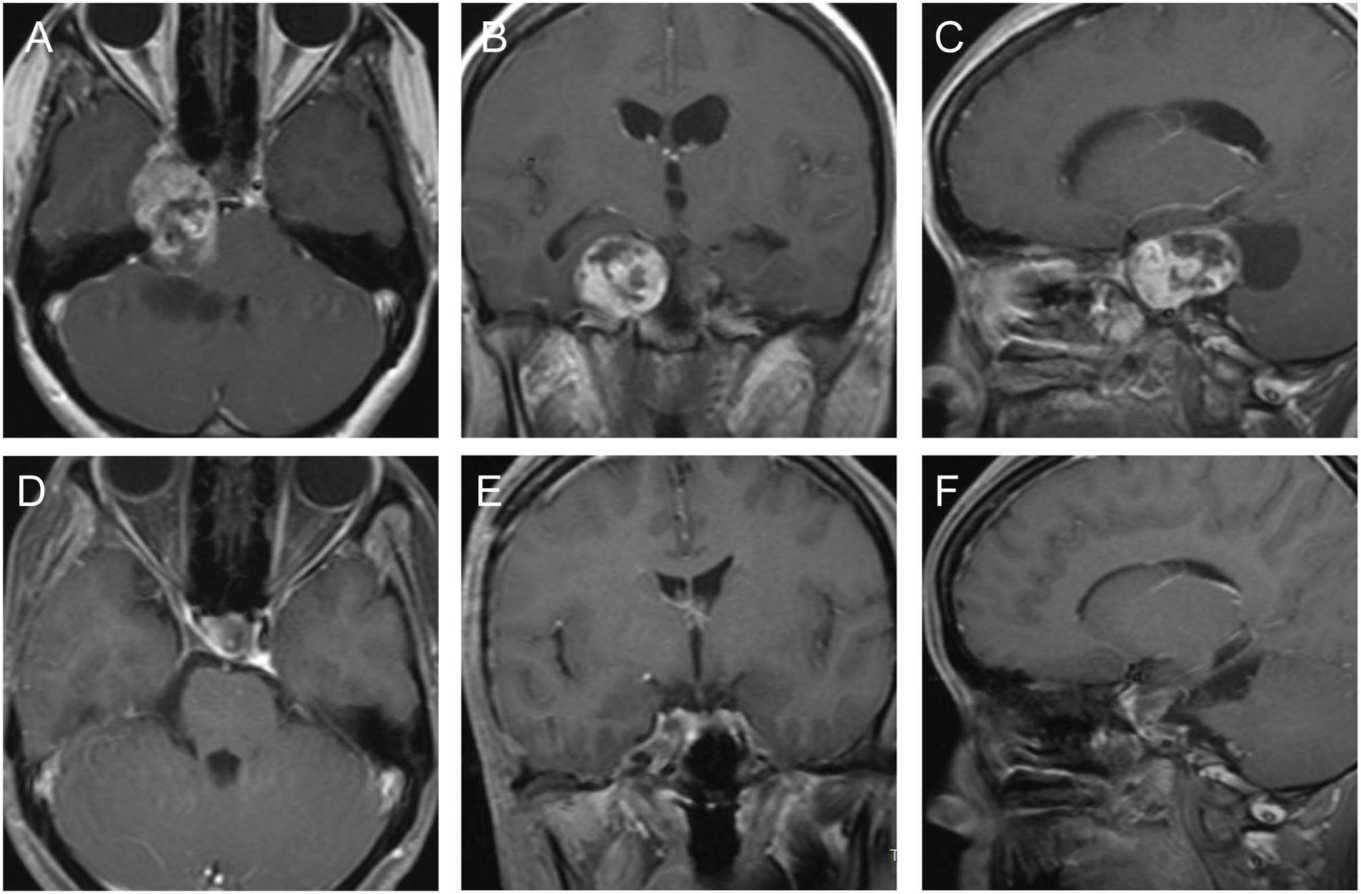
The preoperative and postoperative MRI imaging of case 4. (A–C) Preoperative MRI showed a dumbbell-shaped tumor involving both the right middle and posterior fossae. (D–F) Postoperative MRI showed the most of the tumor was completely removed.
